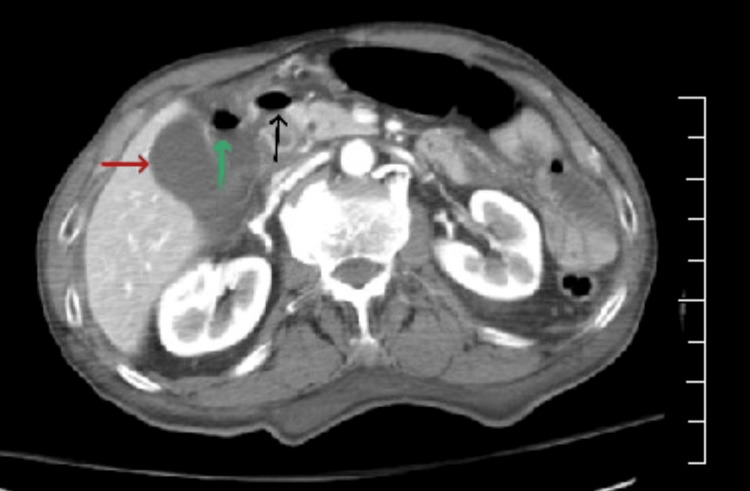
Contrast-enhanced axial CT of the abdomen demonstrating the gallbladder (red arrow) with adjacent extraluminal air (green and black arrows) and fluid in the subhepatic space between the gallbladder and duodenum These findings are highly suspicious for a perforated duodenal ulcer
